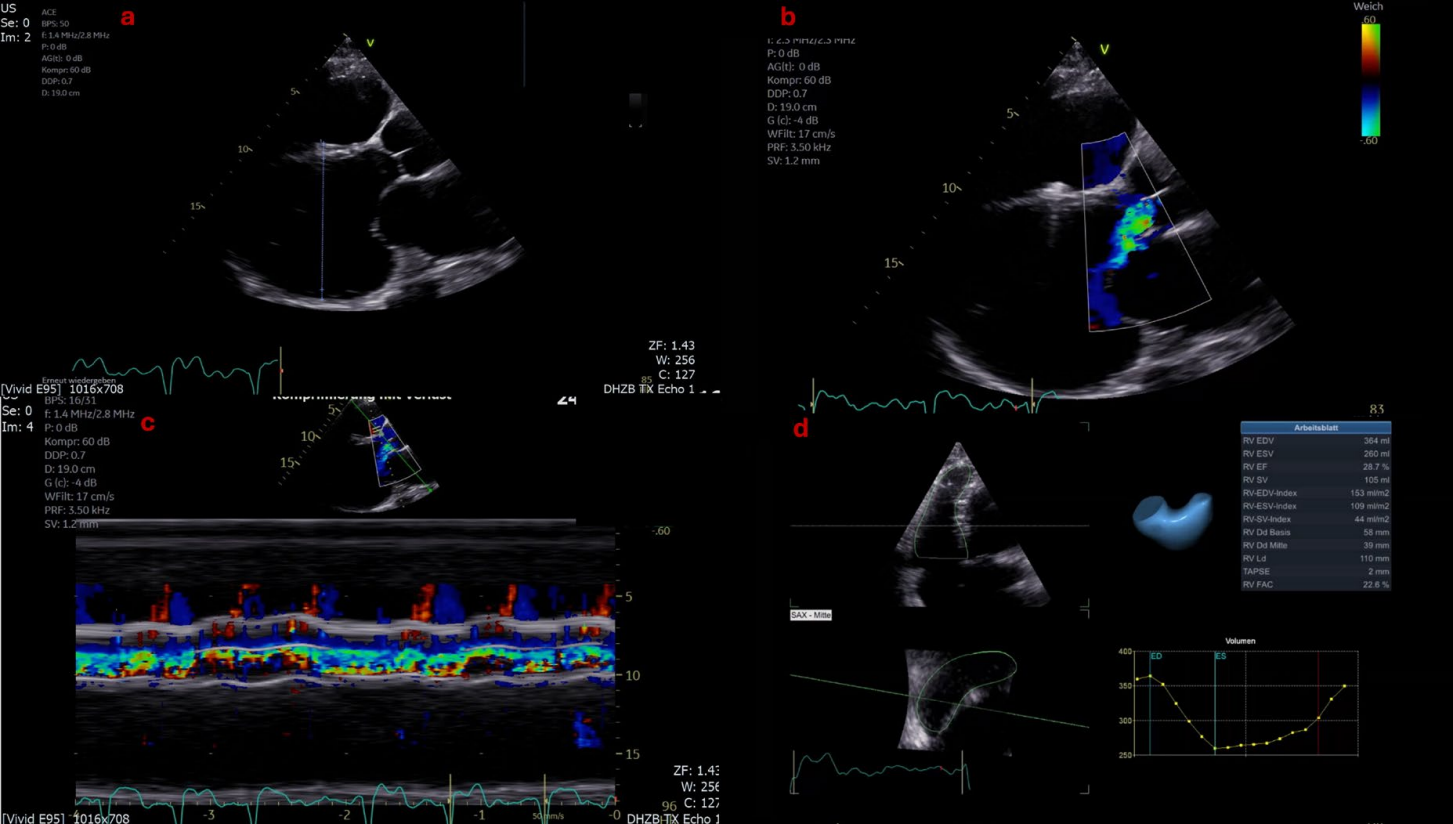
Transthoracic echocardiography: (a) LV dilatation (LVEDD 91 mm) (upper left), (b) significant aortic regurgitation (AR) with eccentric jet toward the mitral valve (upper right), (c) M‐Mode showing diastolic–systolic AR (bottom left), (d) severe RV dysfunction (3D RVEF: 28.7%, FAC: 22.6%) (bottom right). [Color figure can be viewed at wileyonlinelibrary.com]
